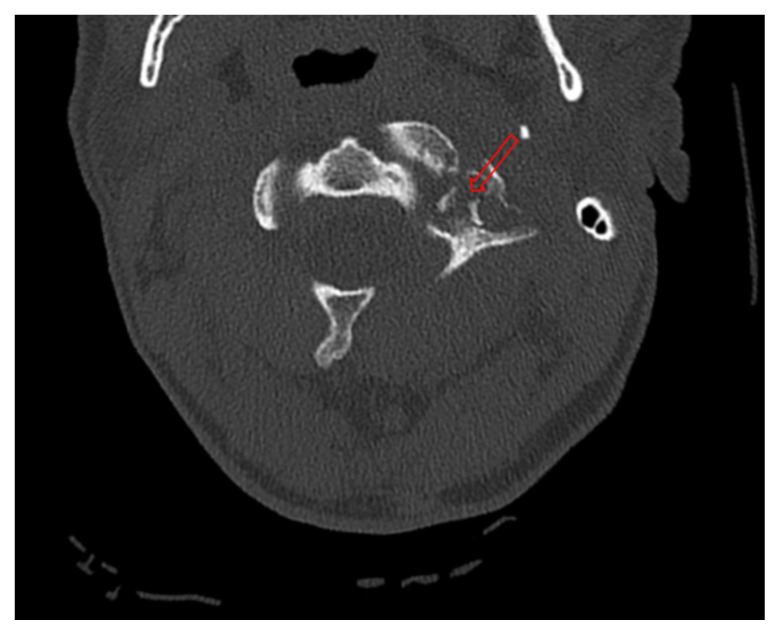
Axial CT Video Link: https://youtu.be/hOogTxYqxto
